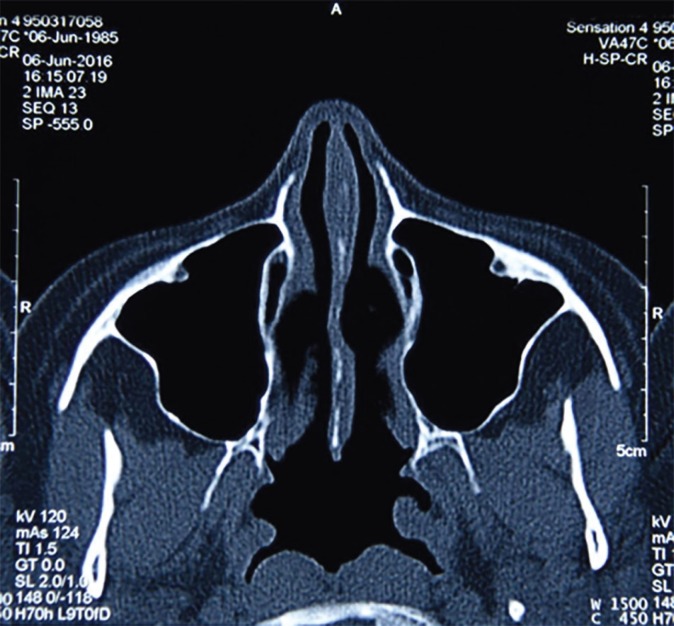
Postoperative axial CT shows corrected septal deviation, open airway, and almost normal view of the area.
